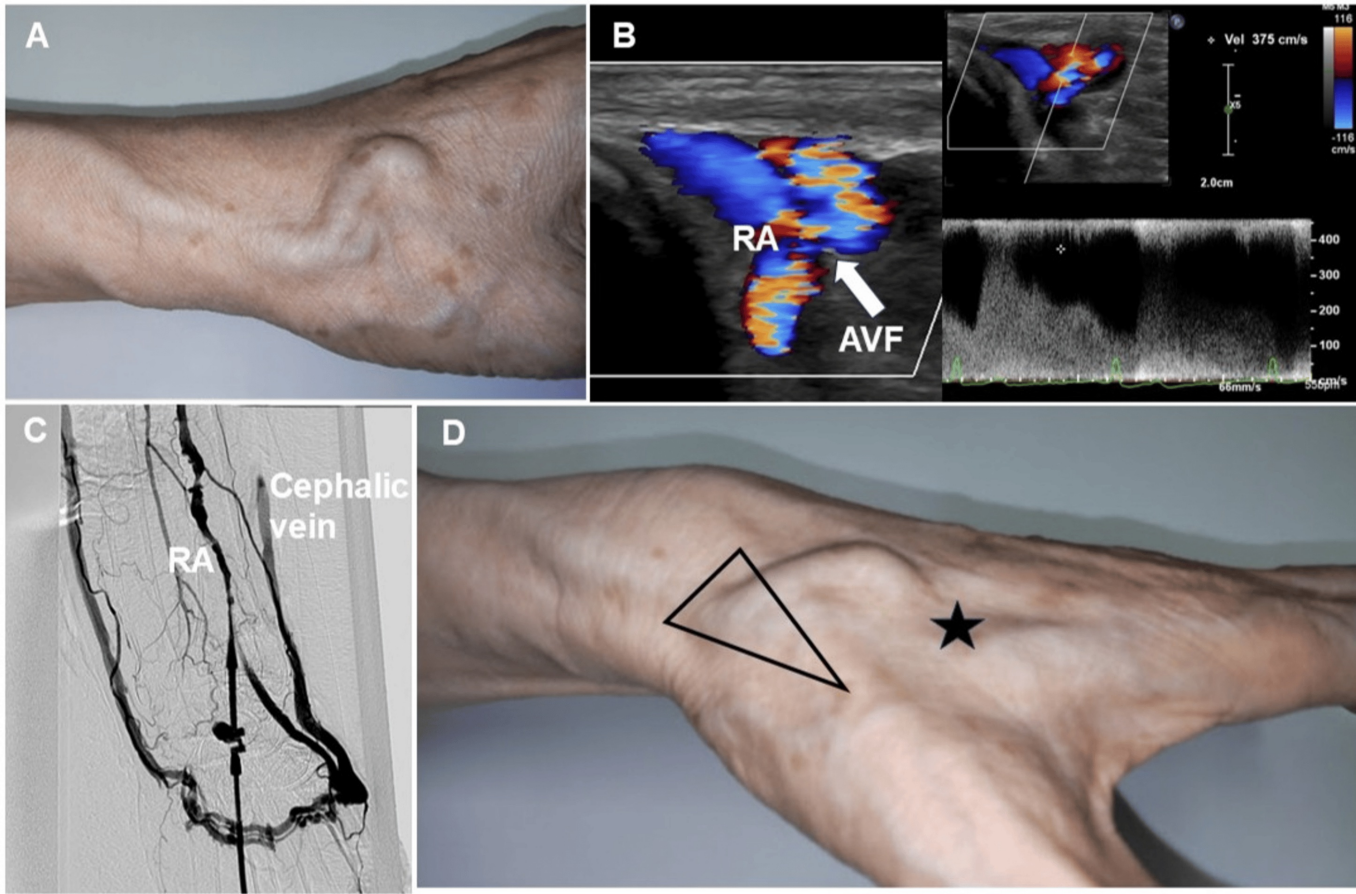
Photographs, echographic findings, and angiogram of arteriovenous fistula formed by distal radial artery puncture. RA: radial artery; AVF: arteriovenous fistula (A) A distended cephalic vein is observed. (B) The arrow shows the entrance to the arteriovenous fistula, where the radial artery connects to the vein. A superficial ultrasonography revealed an arteriovenous fistula in the distal radial artery with a diameter of 2.0 mm and a flow velocity of 3.75 m/sec. (C) Angiography from the radial artery. A shunt was revealed between the distal RA and the dorsal venous network of the hand, and the basilic and cephalic veins were contrasted. It communicates from the radial artery to the vein near the metacarpal bone of the thumb. (D) Common distal radial artery puncture sites (triangle) and the puncture site (star) in this case.
